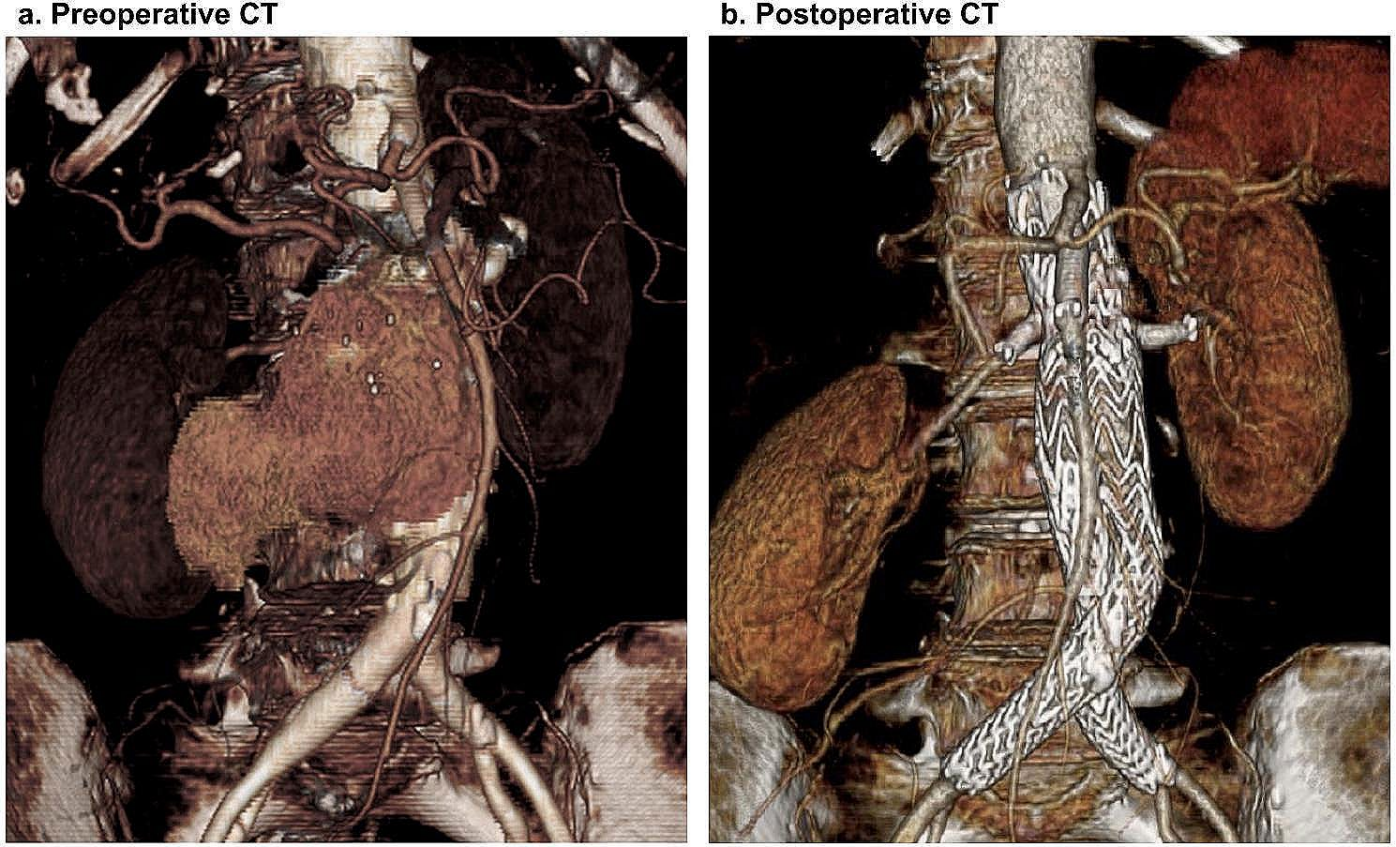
Preoperative and postoperative CT findings a: Preoperative CT shows that the juxtarenal abdominal aortic aneurysm is enlarged toward the right side. b: ChEVAR was successfully performed with preservation of visceral circulation ChEVAR, chimney endovascular aortic repair; CT, computed tomography
